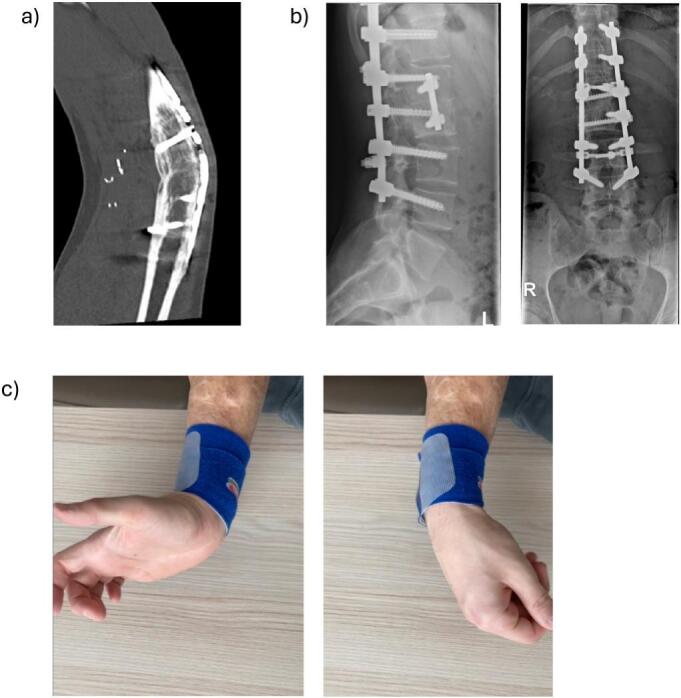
Outcome after 5 years: a) CT-scan of healed elbow-arthrodesis, b) X-ray of healed spinal fracture, c) clinical photography of dorsal and plantarflexion in right wrist.
